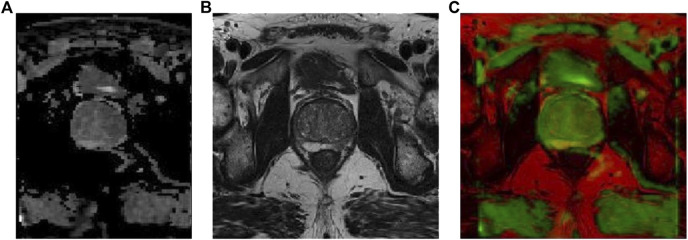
Prostate MR image alignment results, where (A) ADC image, (B) T2-weighted image, and (C) overlap map after image alignment.
